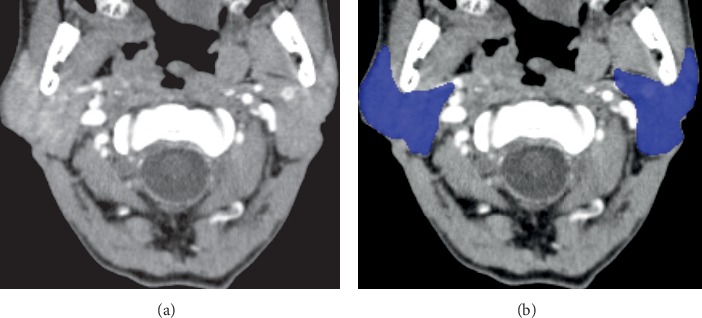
Enhanced-contrast computed tomography (CECT) showing parotid glands structures before chemoradiation therapy (CRT) in the axial slice (a). CECT showing the parotid glands delineation after the segmentation process with the aid of computer software (b).
